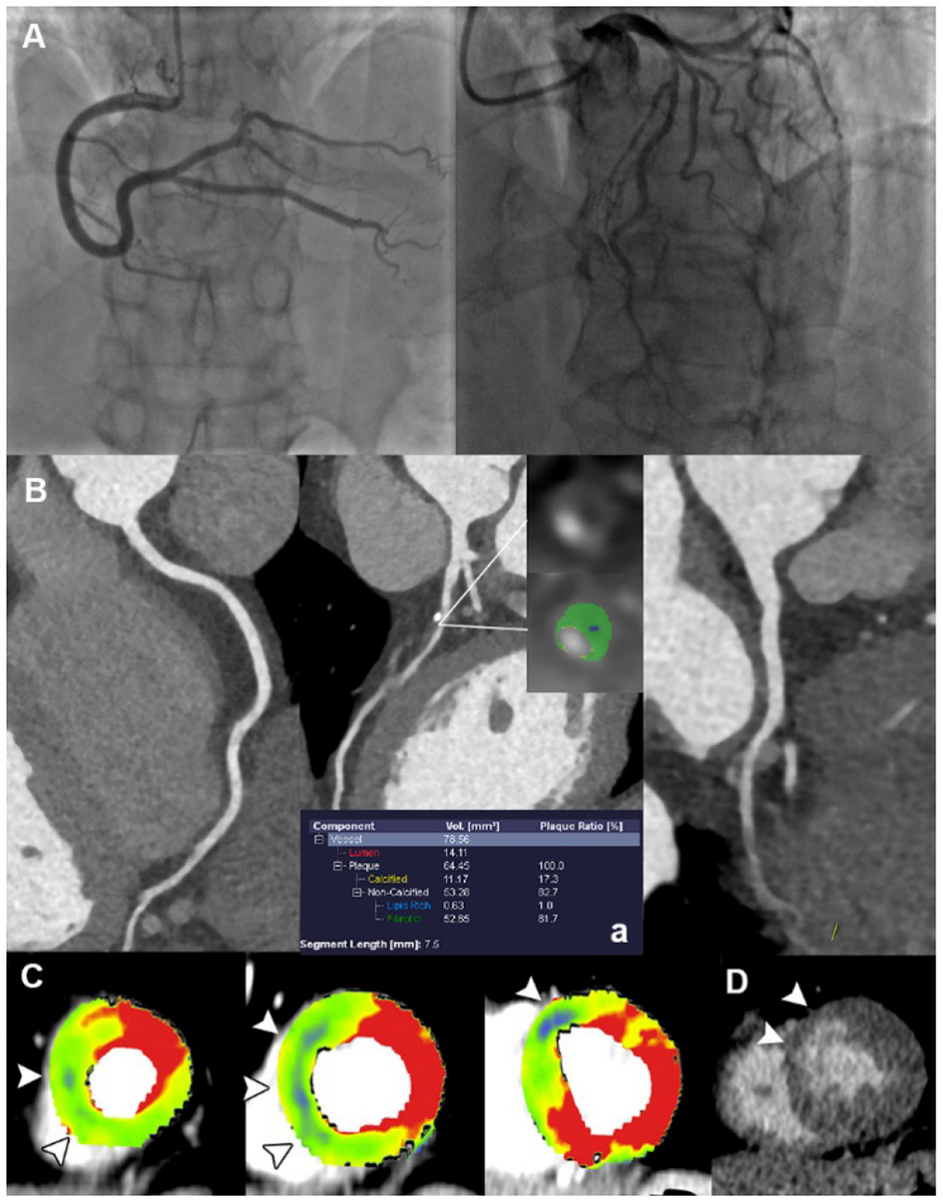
Representative case of plaque disruption in a 61-year-old male with ACS and non-obstructive ICA. (A) ICA revealed mild stenosis at proximal LAD, without presence of obstructive lesion. (B) CPR images showed mixed plaque at proximal LAD with the presence of LAP, PR, NRS, and SC, indicating a typical HRP. (C) Short-axis views of CT-MPI showed significantly reduced myocardial blood flow within LAD territory (white arrowhead). (D) LIE image showed the subendocardial late enhancement within LAD territory (white arrowhead). ACS, acute coronary syndrome; CPR, curved planar reformation; CT, computed tomography; ICA, invasive coronary angiography; LAD, left anterior descending; LAP, low-attenuation plaque; LIE, late iodine enhancement; MPI, myocardial perfusion imaging; MVO, microvascular obstruction; NRS, napkin-ring sign; PR, positive remodeling; SC, spotty calcification.
